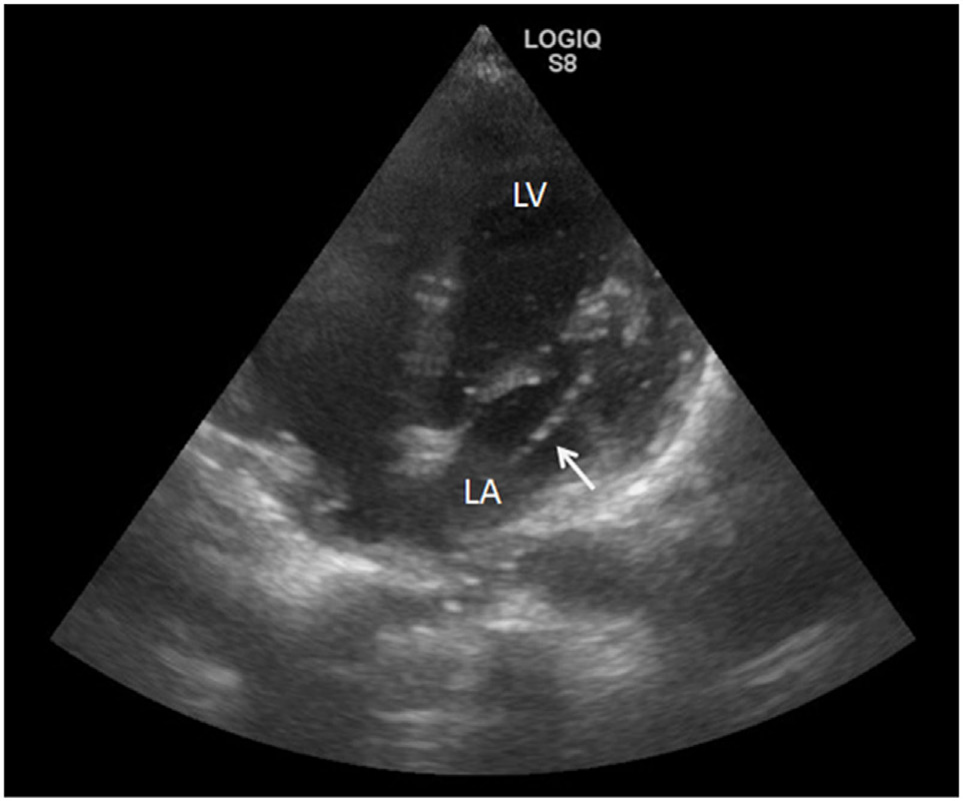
Heart ultrasound showing the misplacement of a central venous access device (white arrow) *via* the interatrial septum, the left atrium (LA), and the mitral valve up to left ventricle (LV) during echo bubble test.
